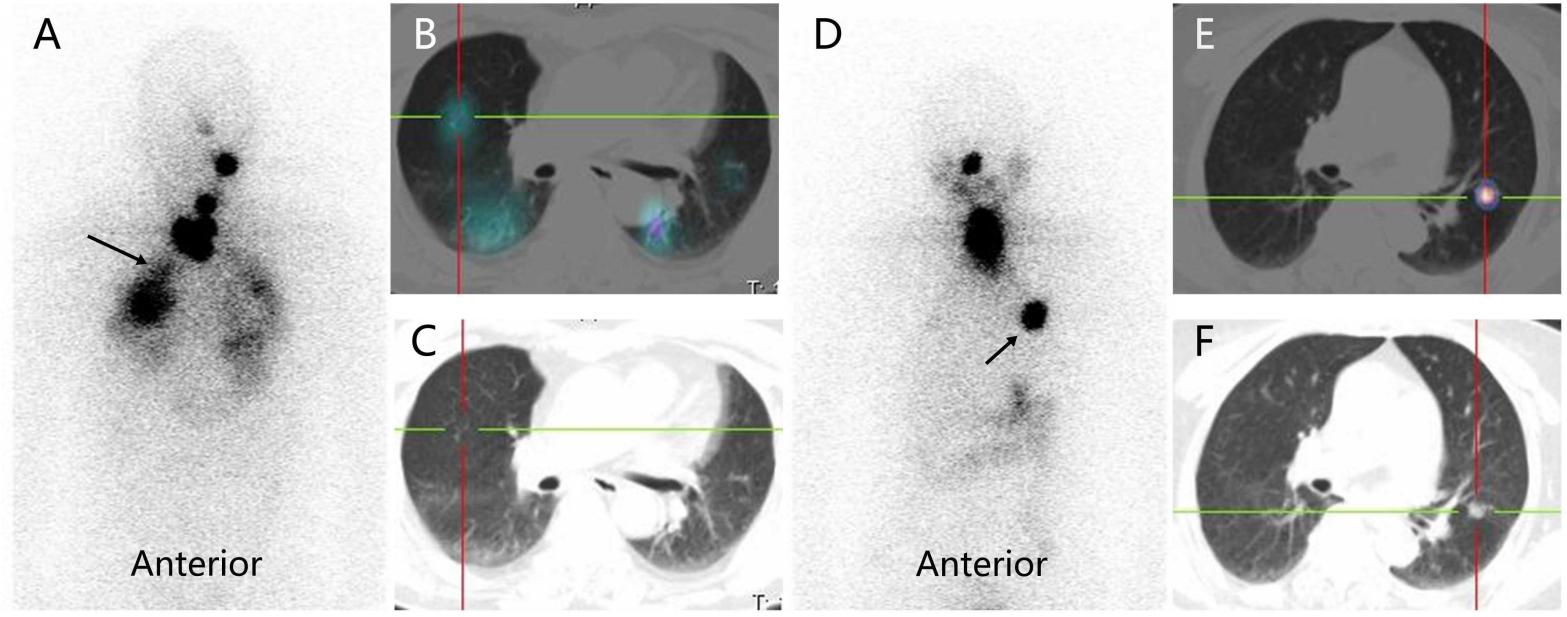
RAI-avid lung metastases from differentiated thyroid cancer in a male patient (RAI-avid DTC-LM lesions are visible only on Rx-WBS but not on chest CT) and a female patient (RAI-avid DTC-LM lesions are visible both on Rx-WBS and chest CT). (A, D) anterior Rx-WBS; (B, E) SPECT/CT fusion images post initial course of RAI therapy; (C, F) CT images post initial course of RAI therapy; RAI, radioiodine; DTC, differentiated thyroid cancer; LM, lung metastasis; Rx-WBS, therapeutic ^131^I whole-body scan.
